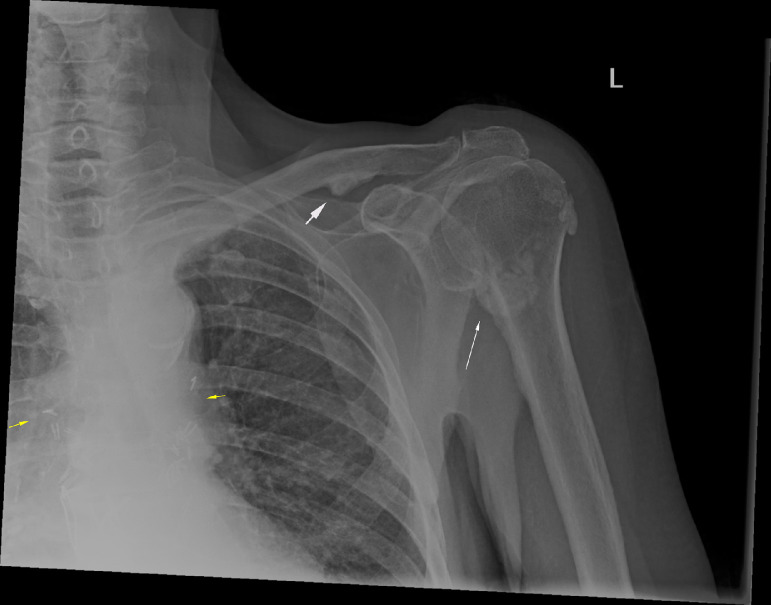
AP radiograph of the left shoulder: There are foci of juxta cortical mineralisation at the proximal left humerus (white arrow) and inferior left clavicle (white arrowhead) consistent with periostitis. Surgical clips are noted at the hila bilaterally (yellow arrows) in keeping with the history of bilateral lung transplantation.
